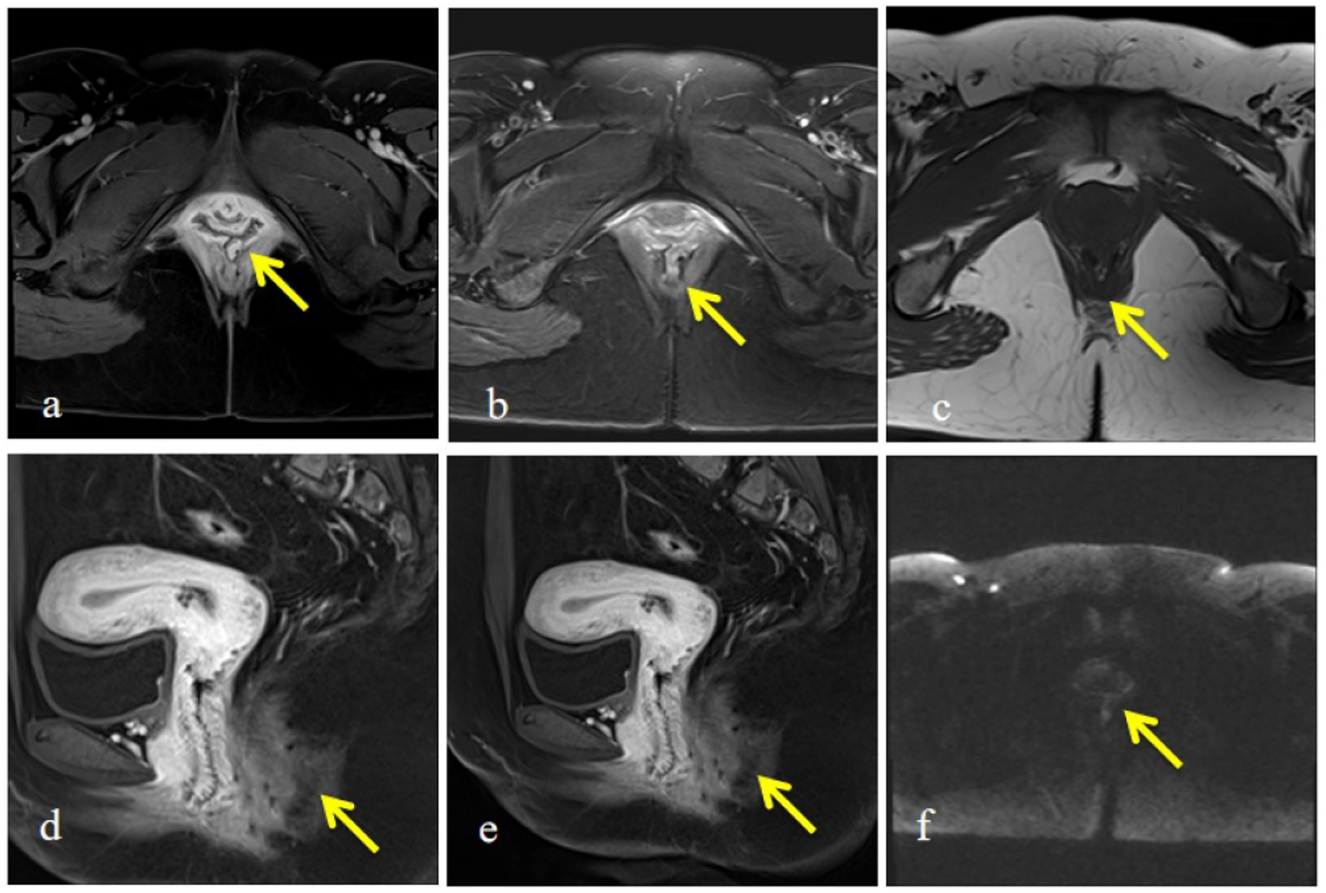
Pelvic magnetic resonance imaging demonstrating rectovaginal fistula. (a) Contrast-enhanced axial image showing disruption of the rectovaginal septum with abnormal communication between the rectum and vagina (arrow). (b) T2-weighted axial image demonstrating hyperintense fistulous tract connecting the rectum and vaginal lumen (arrow). (c) T1-weighted axial image revealing structural discontinuity of the posterior vaginal wall (arrow). (d) Contrast-enhanced sagittal image showing fistula tract extending from the rectum to the posterior vaginal wall (arrow). (e) Sagittal contrast-enhanced image further delineating the fistulous pathway and surrounding soft tissue changes (arrow). (f) Diffusion-weighted imaging highlighting the fistulous region with abnormal signal intensity (arrow).
